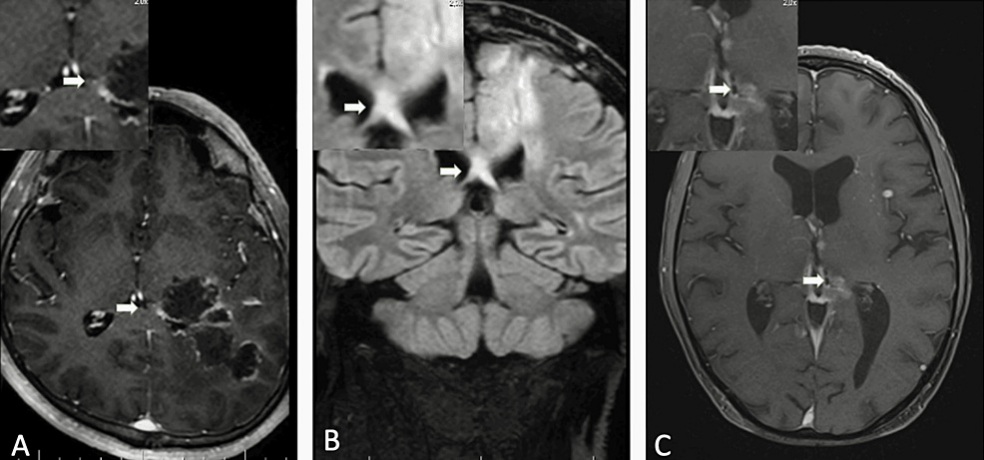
Primary tumors (A) Post-contrast T1-weighted image showing recurrent lesion invading the left crus of the fornix (arrow) in a 56-year-old male diagnosed with glioblastoma. (B) Post-contrast fluid-attenuated inversion recovery (FLAIR) image showing lesion crossing midline via the corpus callosum and invading the bilateral crus of the fornix (arrow) in a 45-year-old male diagnosed with diffuse astrocytoma. (C) Post-contrast T1-weighted image showing recurrent lesion invading both the crus and the body of the left fornix (arrow) in a 25-year-old male diagnosed with medulloblastoma. 2.0× magnified images of the lesions are shown in the upper left corner.
